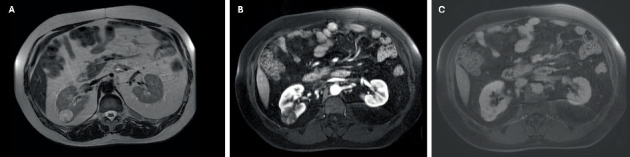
(A) MRI of the abdomen T2 axial image shows internal heterogeneity of the 3.6 cm Bosniak 4 posterior right renal mass more clearly. Axial postcontrast T1 fat-saturated images show (B) early and (C) late enhancements of the mass. Areas of solid enhancement become increasingly visible on late postcontrast imaging.
